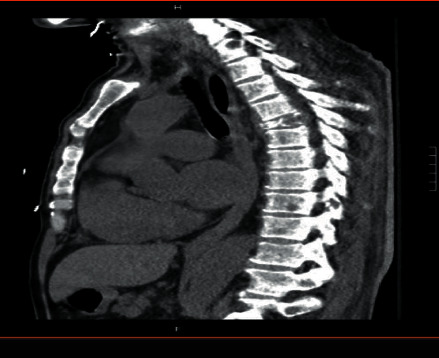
CT scan showing lytic bone lesions.
